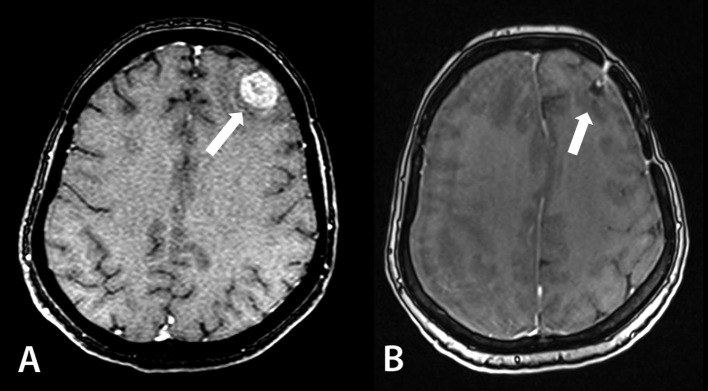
MR images of patient 1 before combined treatment (A) and after 12 months of follow-up (B). No radiation-induced toxicity in the metastatic tumor bed in the left frontal lobe is noticed. MR: magnetic resonance
